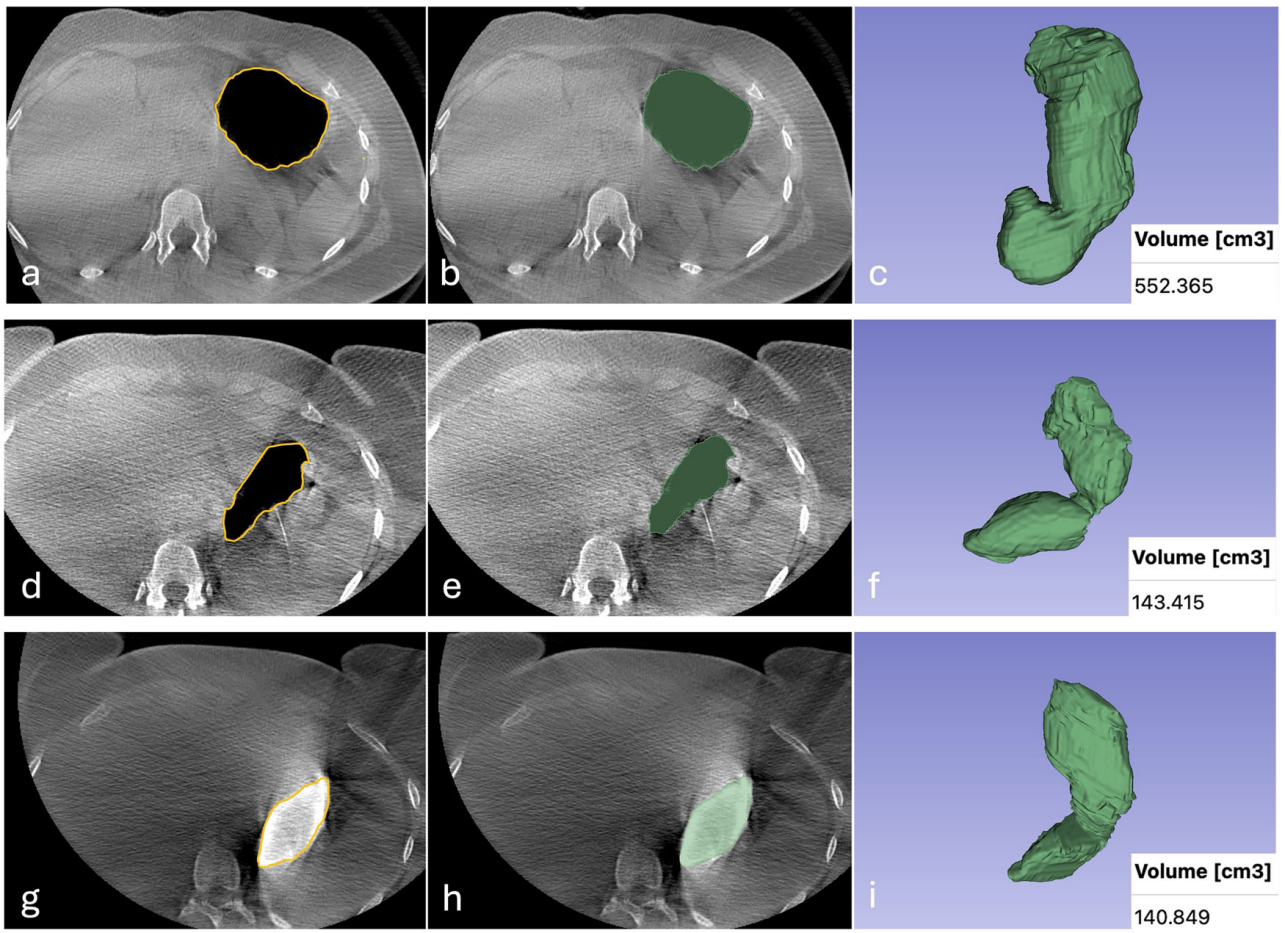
Upper abdomen CBCT images in a 45-year-old woman with a BMI of 32 kg/m^2^. Manually drawn regions of interest of the stomach with CO_2_ gastric insufflation before (a) and after ESG (d). Manually drawn region of interest of the stomach with administration of a diluted Gastrografin solution after ESG (g). The same images showing the stomach after volumetric segmentation (in green; b, e, h). The same stomach in 3D rendering (c, f, i). CBCT, Cone-beam computed tomography; ESG, Endoscopic sleeve gastroplasty
